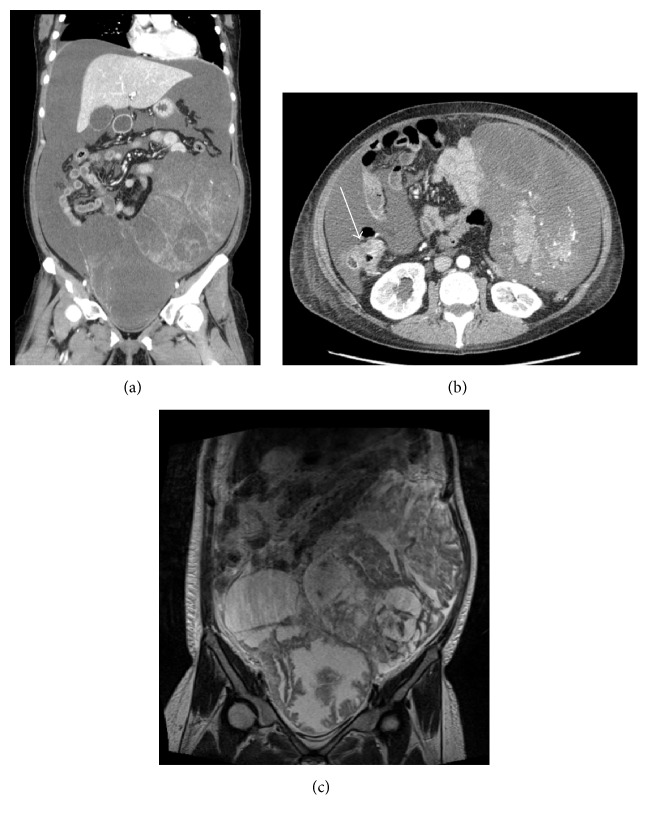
Case  2. Contrast-enhanced CT scan shows a well-circumscribed and heterogeneously enhanced tumor of the ovary ((a) coronal plane) and wall thickening of the ascending colon with enhancement (arrow; (b) axial plane). MRI T2 shows cystic masses in the pelvis ((c) coronal plane).
